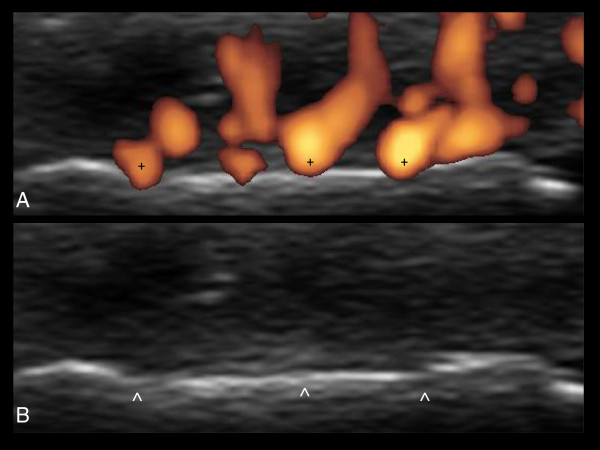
Early aggressive arthritis (dorsal view, transverse scan). The Doppler signal is closely linked to the bone margin (A). The same image without Doppler signal (B) shows an evident circumscribed loss of sharpness of the bone margin (>) at the point of contact with the synovial pannus (+). This figure is original and has not been previously published.
